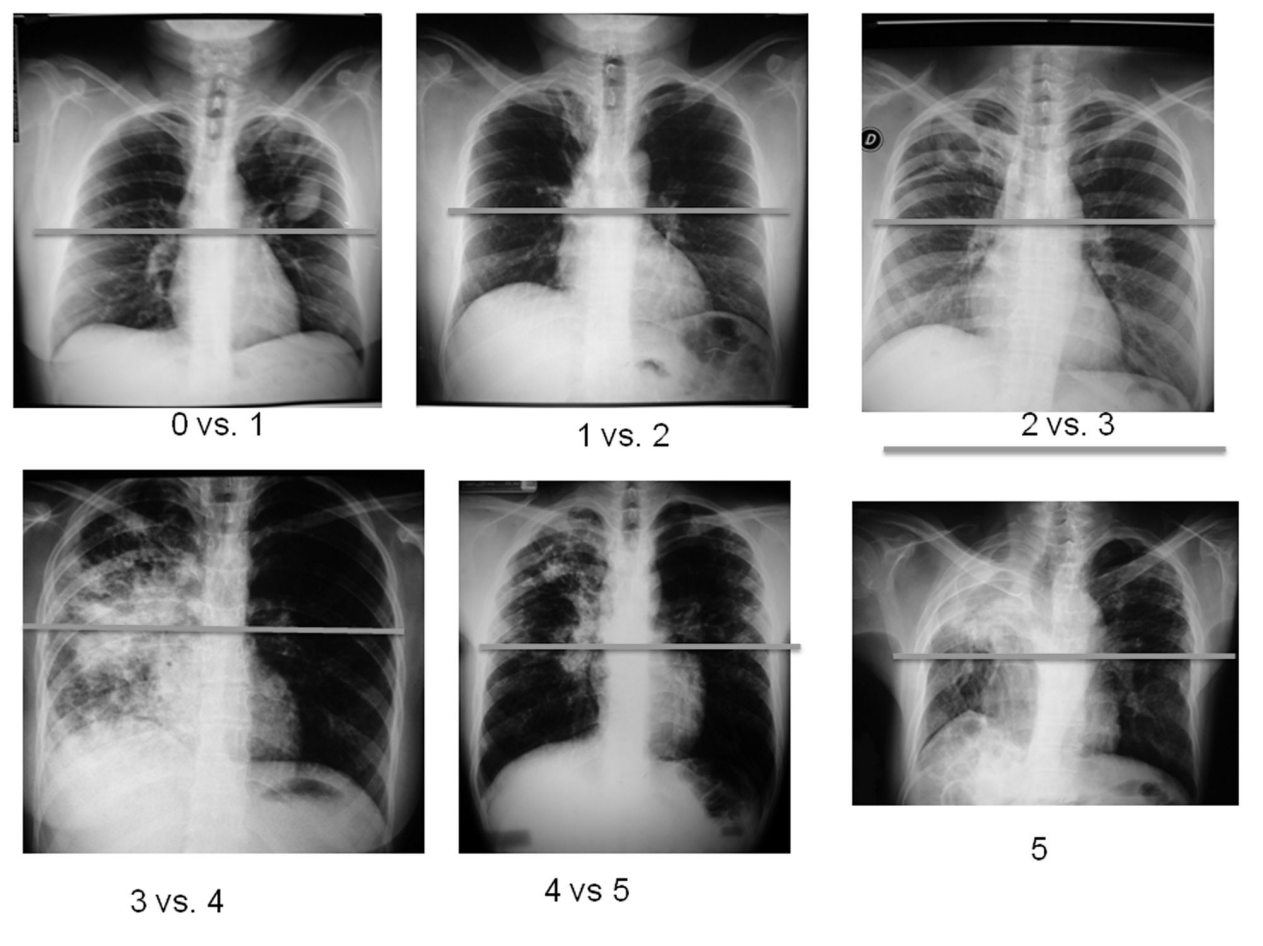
Representative example radiographs of quadrants for each score, according to the degree of radiographic abnormality using the same quadrant (upper left). The radiographs are from different patients from the study.
